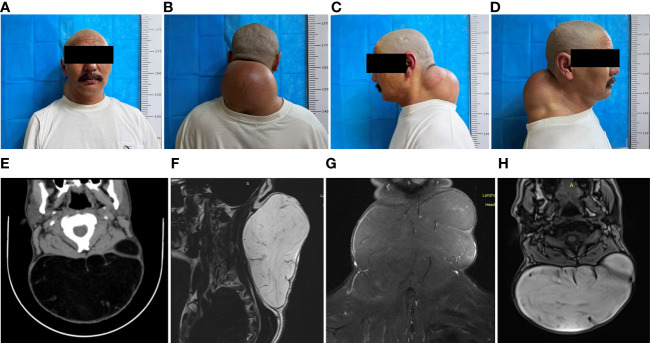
Preoperative clinical and imaging presentation of a giant liposarcoma of the neck. (A) Preoperative anterior section. (B) Preoperative dorsal view. (C, D) Preoperative lateral views. (E) Preoperative CT of the neck shows a large posterior cervical mass with clear borders, measuring approximately 162 × 92 × 166 mm, with a CT value of approximately -108 HU. Separation shadows are seen within the mass. (F–H) MRI of the cervicothoracic segment showed a large cervical mass with a double-high signal, reduced T2-fs signal, and a linear separation signal shadow in the neck; a linear enhancement shadow was seen on DWI. Cervicothoracic MRI showed a large cervical mass with double high signals in the cervical spine, reduced T2-fs signals, and linearly separated signal shadows, measuring approximately 166×17.6×10.7 cm, with low signals on DWI and linear enhancement on the enhancement scan. The cervical spine was normal with physiological curvature; a mass-like T2-FS high signal shadow was seen in the spinal cord at the C2 level.
